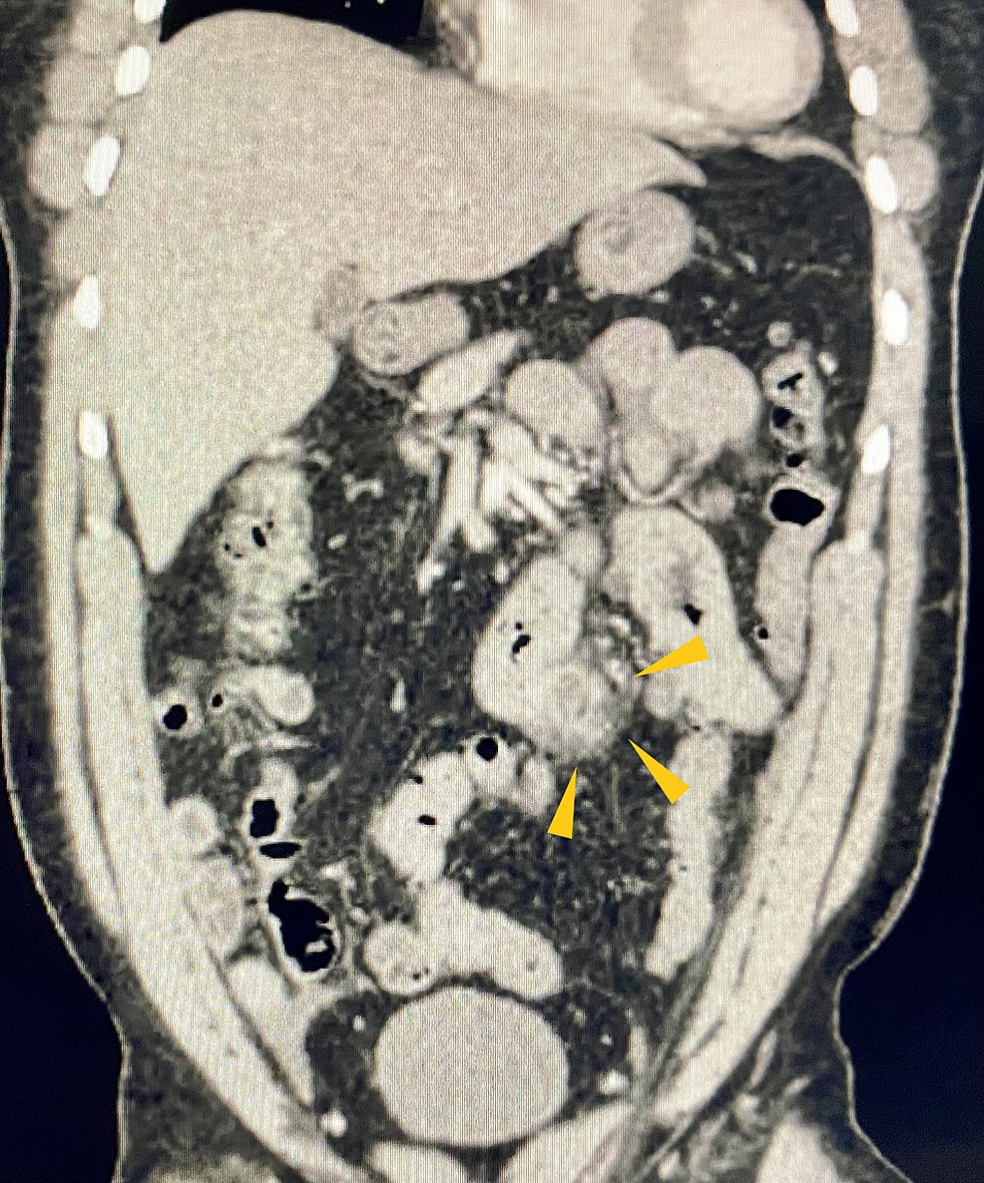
CT of the abdomen and pelvis with contrast (coronal view). Showing multiple diverticula (yellow triangle) involving the jejunum.
